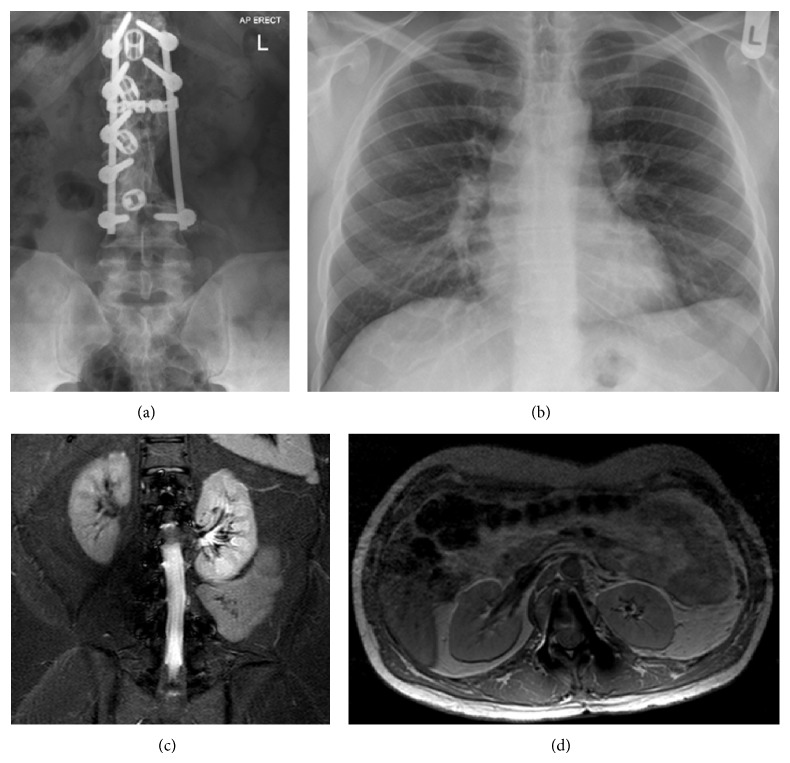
Five-year follow-up imaging (a) anteroposterior lumbar radiograph demonstrating stability of instrumentation. (b) Posteroanterior chest radiograph with clear lung fields. (c and d) Axial MRI at the level of L1 and coronal MRI with no evidence of macroscopic local tumour recurrence.
